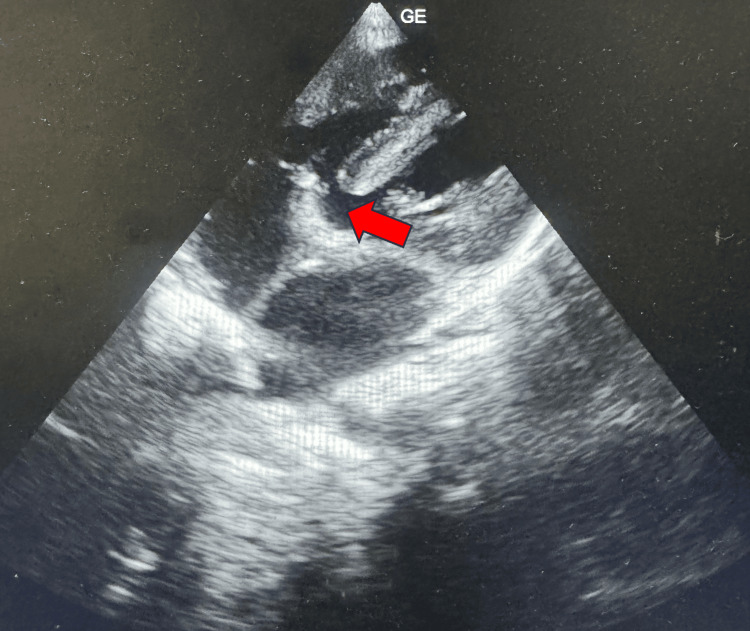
2D echocardiography showing ventricular septal defect (red arrow)
